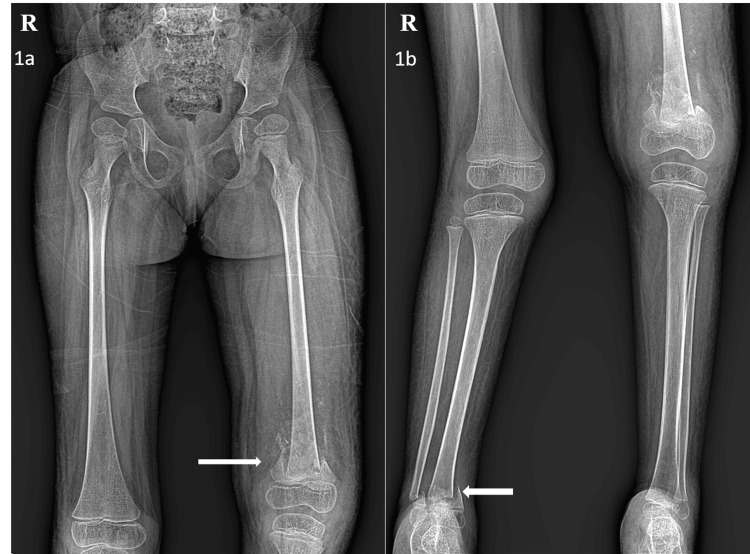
Antero-posterior view radiographs of the pelvis with both hips and the bilateral femur with knee joints (1a) and bilateral legs with ankle joints (1b), showing generalized decreased bone density with fracture of the distal metaphysis of the left femur with callus formation and fracture of the distal metaphyses of the right tibia and fibula (white arrows)
